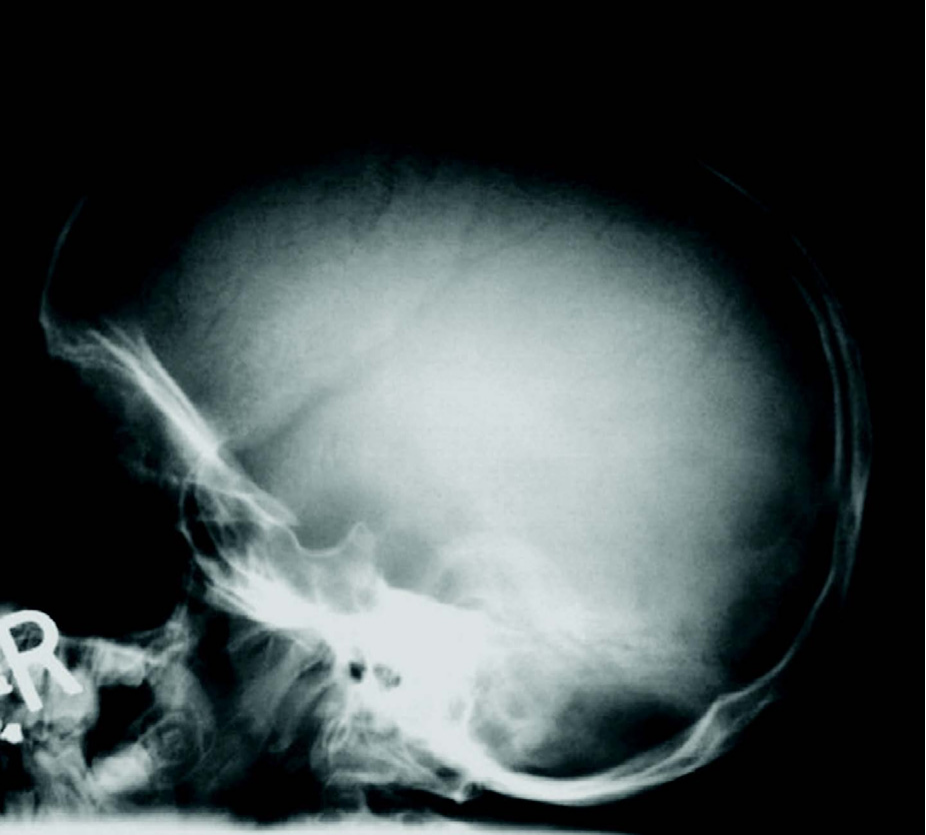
Rapidly progressing 5-year old female MPS VI patient: radiograph of cranium showing thickened diploic space and J-shaped sella.
